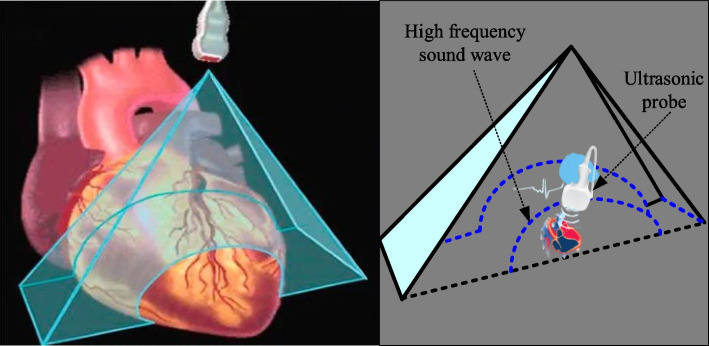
Principle of three-dimensional echocardiography
